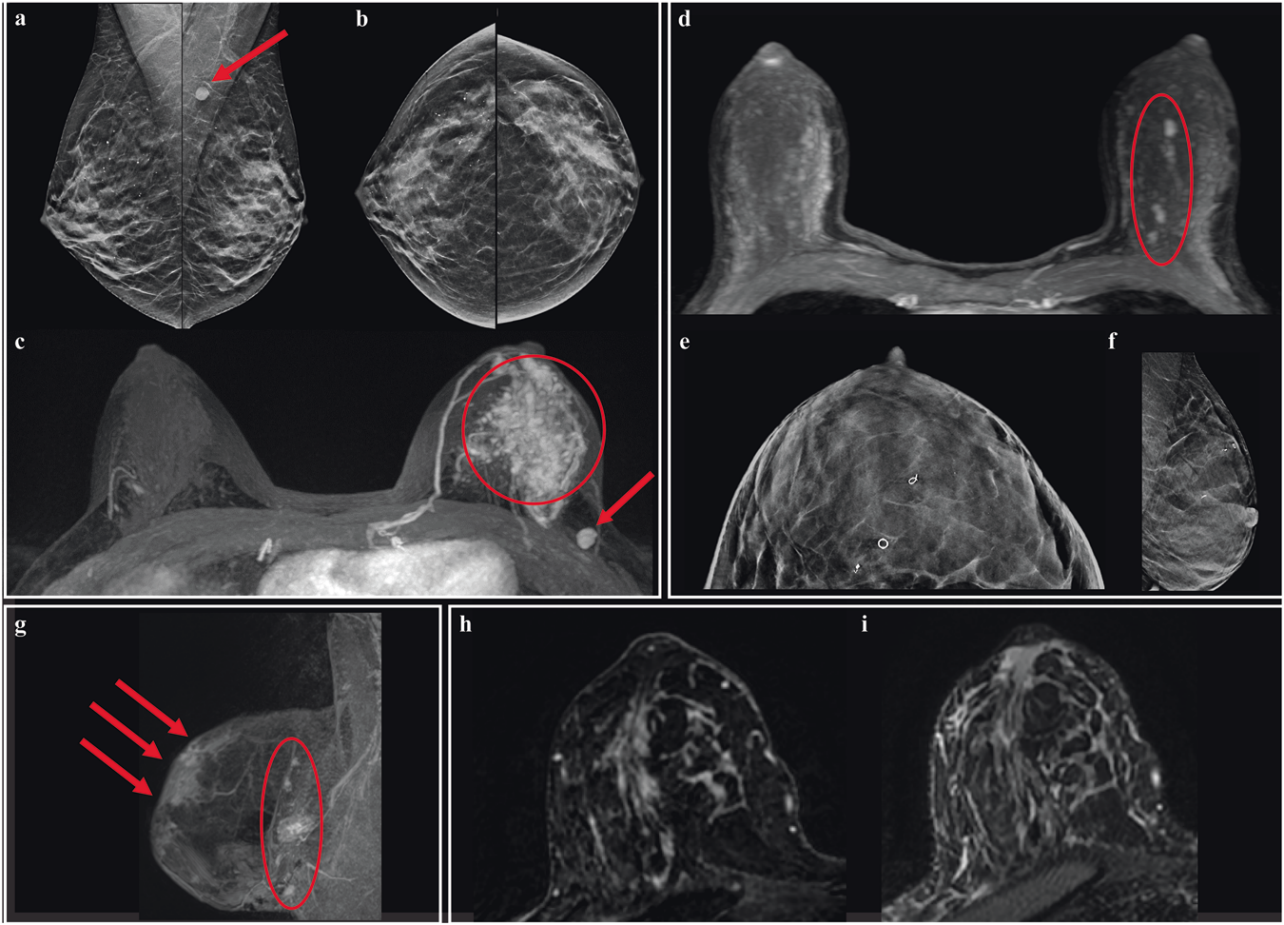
Breast imaging in women with hereditary lobular breast cancer. Patients with HLBC undergoing diagnostic breast MRI either due to a palpable abnormality or an abnormality detected on a screening mammogram generally had a significantly larger tumor burden at the time of diagnosis compared with patients whose breast cancer was diagnosed on screening breast MRI. In a 47-year-old female with an unknown germline *CDH1* variant presenting with a palpable abnormality, a mammogram demonstrated subtle asymmetry in the left breast on both mediolateral (a) and craniocaudal (b) views. Follow-up imaging with breast MRI axial MIP (maximum intensity projection) (c) showed an approximate 7-cm region of non-mass enhancement (red circle) and a prominent left axillary node with rounded morphology, which was also seen on the original mammogram (red arrow). Final pathology demonstrated a 7-cm mixed ductal/lobular carcinoma in both the left upper outer quadrant and the left lower outer quadrant. In contrast, a 45-year-old woman with known germline *CDH1* P/LP variant received a recommended screening breast MRI (d), which demonstrated multiple small enhancing masses in the left breast, seen on an axial MIP image (red circle). Follow-up ultrasound and biopsy with clip placement confirmed two sites of malignancy in the left breast. Post-biopsy mammogram assessing clip placement sites demonstrated dense breast tissue without an obvious mass at biopsy sites on either the mediolateral (e) or craniocaudal (f) views. The final pathology for this individual demonstrated a 1.1-cm invasive lobular carcinoma. To showcase MRI detection of uncommon features, (g) depicts a 66-year-old female with known germline *CDH1* P/LP variant originally diagnosed with a 6-cm invasive lobular breast carcinoma at age 65 who was treated with bilateral mastectomy and systemic chemotherapy but developed skin changes the following year; follow-up breast MRI demonstrated several prominent intramammary and axillary lymph nodes (red circle, sagittal view) with subdermal enhancing abnormalities and skin thickening signaling recurrence of disease (red arrows). Last, techniques such as STIR may further assist in the detection of tumors on breast MRI, as seen in Figures h, i: in this case, a 52-year-old female with a known germline *CDH1* variant demonstrated an ill-defined large infiltrating tumor in the right breast on post-contrast axial MRI (h), correlating with parenchymal edema on corresponding STIR sequence (i).
